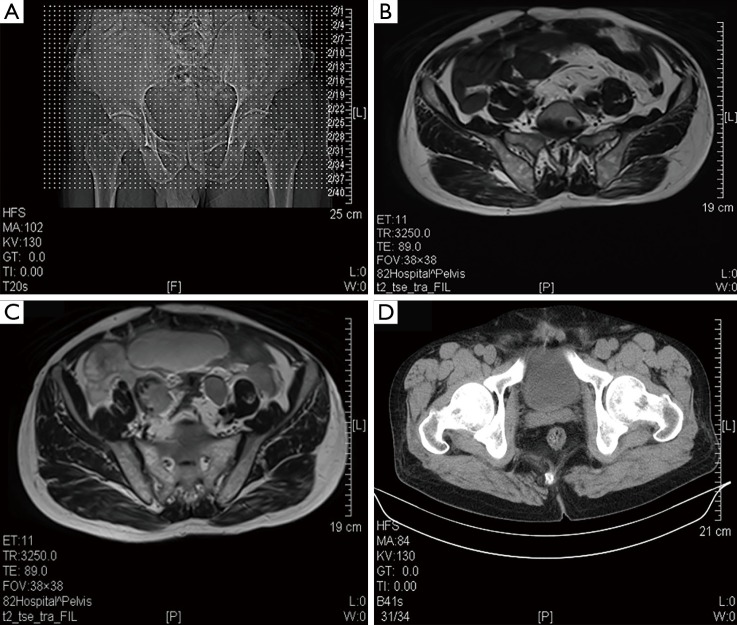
Three abdominal CT images taken 6 months after the last cycle of EP chemotherapy showed no signs of HPC recurrence. CT, computerized tomography; HPC, haemangiopericytoma.
